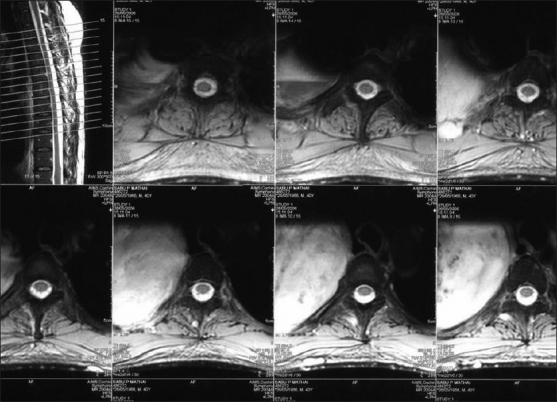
MRI of the thoracic spine sagittal T1 T2 level showing a large mass in the right thorax of which the medial border is adjacent to the thoracic vertebra. No evidence of vertebral encasement or intraspinal extension seen
